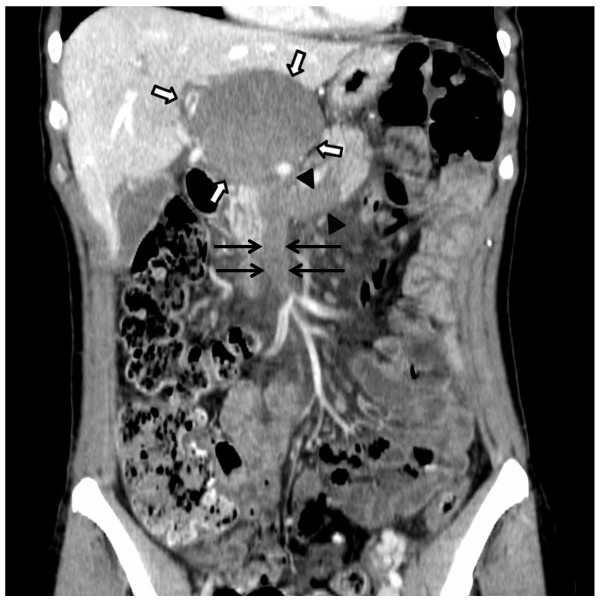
CT-scan showing thrombosed portal vein aneurysm (white arrows) with thrombus extending to SMV (black arrows) and splenic vein (arrowheads).
